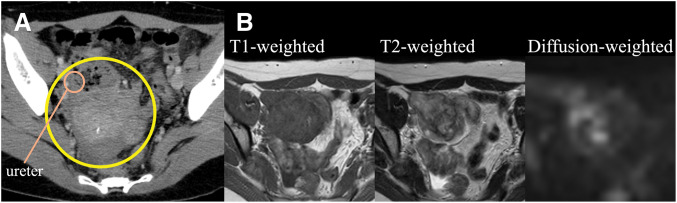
Preoperative imaging. (A) Contrast-enhanced CT revealed a 67-mm mass in the cecum with irregular wall thickening, heterogeneous enhancement, and encapsulated fluid collection (yellow circle). A linear high-density area within the mass suggested the presence of an appendiceal fecalith. The right ureter was closely adjacent to the mass (orange circle). (B) MRI revealed cecal wall thickening that appeared hypointense on T1-weighted images (left image), slightly hyperintense on T2-weighted images (center image), and partially hyperintense on diffusion-weighted images (right image).
